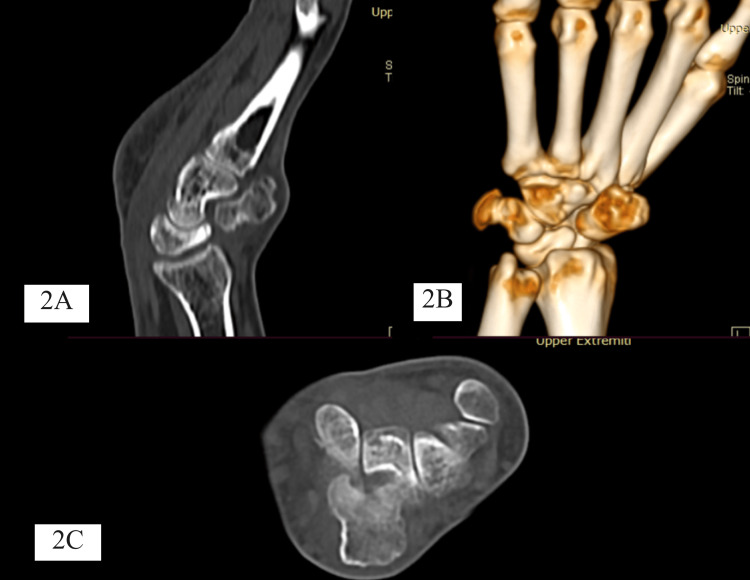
Pre-operative sagittal (A), 3D image (B), and axial cut sections (C) of computed tomography images depicting a left wrist bony lesion arising from lunate bone
